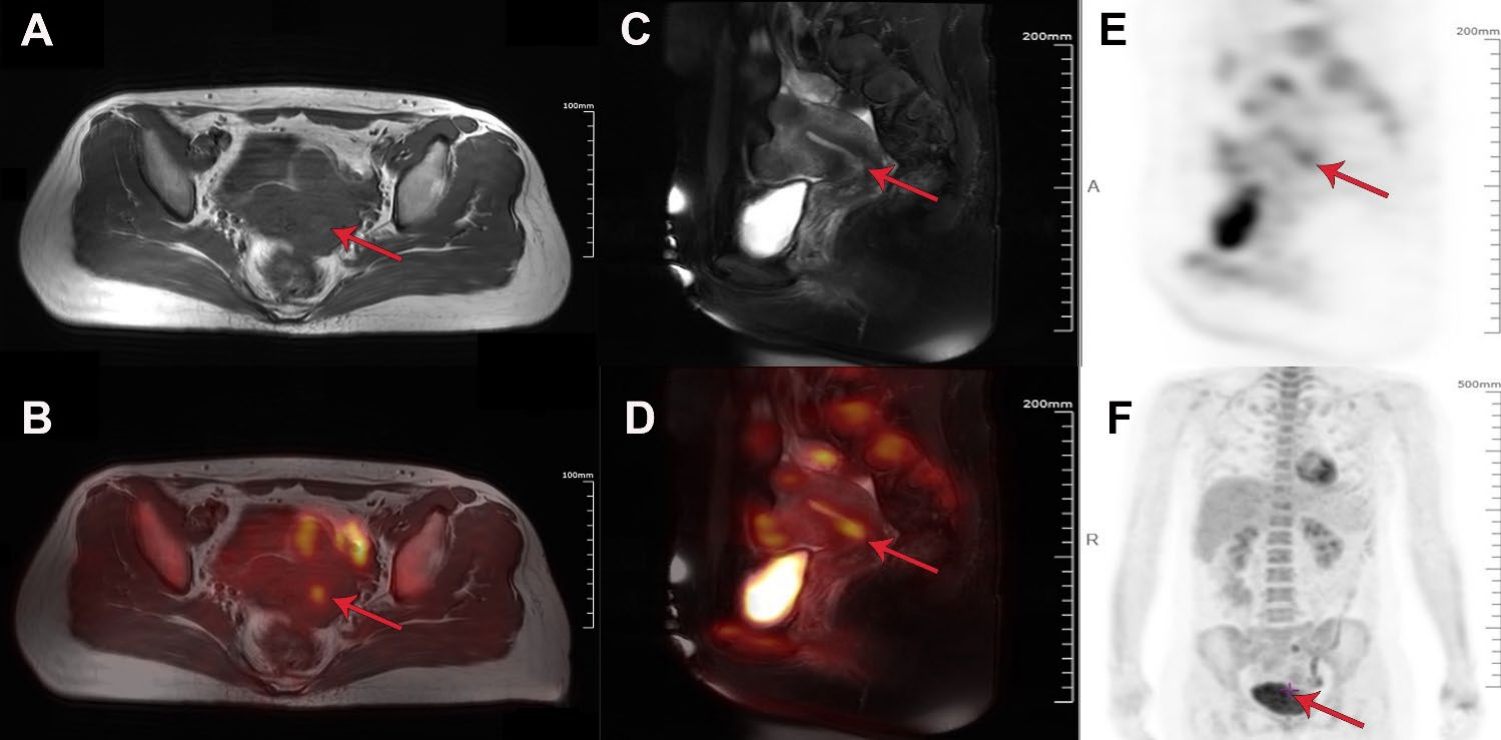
Positron emission tomography-magnetic resonance imaging (PET-MRI) of the ES patient. A–B An axial T2-weighted MRI image and an axial FDG PET-MRI fused image. C–D A coronal T2-weighted MRI image and a coronal FDG PET-MRI fused image. E–F Coronal FDG PET images performed simultaneously with MRI anterior and right. Increased FDG metabolism in the anterior wall of the cervical segment of the lower uterine body (red arrows)
